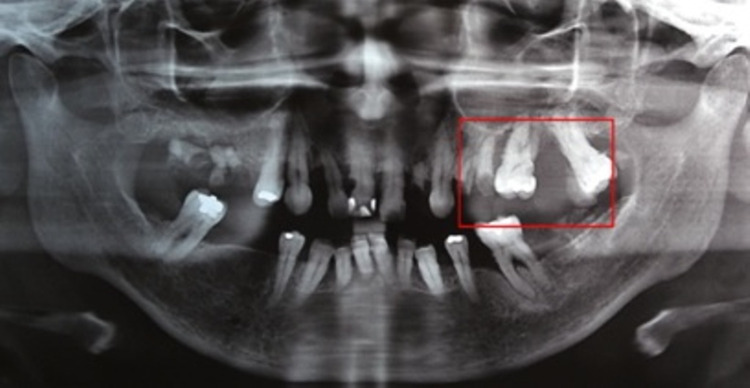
Panoramic radiograph showing a radiopaque image associated with the left posterior maxillary alveolar process, with divergence of the crowns of the first and second molars and loss of bone insertion.
